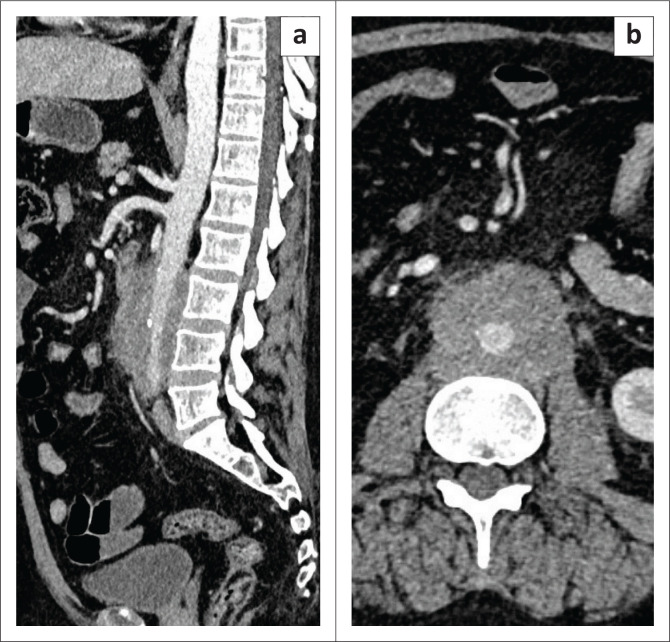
Sagittal reformatted (a) and axial (b) sections of the contrast-enhanced CT abdomen of case 2 demonstrate a fibrotic soft tissue cuff encasing the abdominal aorta with no definite luminal effacement of the aorta.
